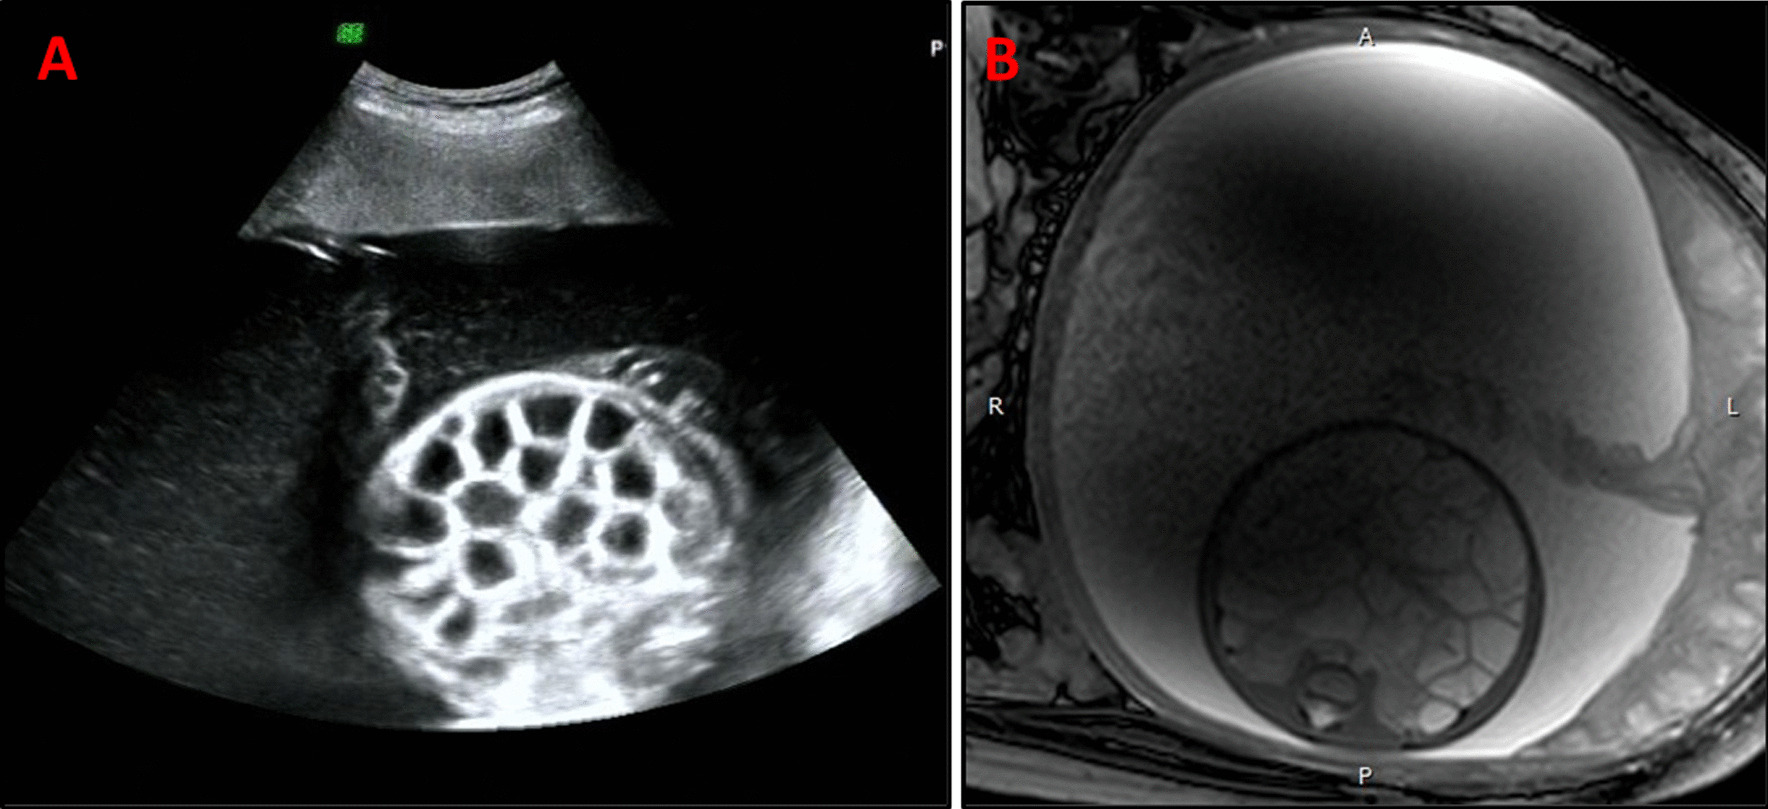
Prenatal imaging of Patient 3. A Dilated loops of fetal bowel and polyhydramnios were visible by ultrasound at 24 weeks’ gestation. B MRI scanning confirmed the presence of polyhydramnios and fetal diffuse intestinal dilation at 26 weeks’ gestation
